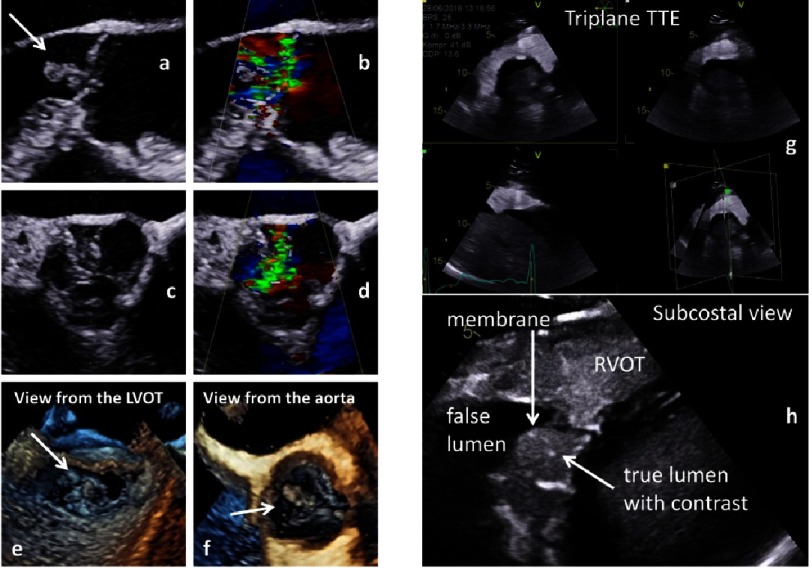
Documentation of vegetations due to endocarditis in native and color-coded 2D transthoracic long axis views (a, b) and 2D transesophageal short axis views (c, d) as well as 3D transesophageal en-face views of the AV from the LVOT (e) and the tubular ascending aorta (f). On the right side aortic dissection (Stanford A) is documented in a triplane subcostal view using contrast echocardiography (g) and in a zoom view of the dissection membrane (h).
